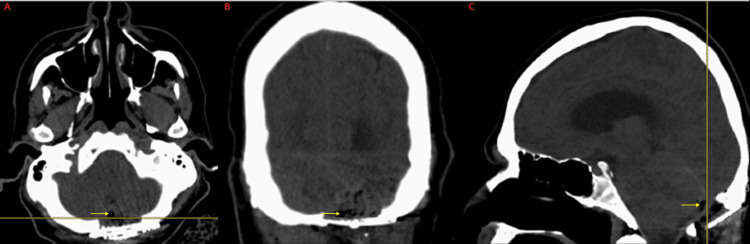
Immediate postoperative CT of the head without contrast in the axial plane (A), coronal plane (B), and sagittal plane (C) A small amount of pneumocephalus is present in the surgical cavity (yellow arrows), which is an expected postoperative finding CT: computed tomography
